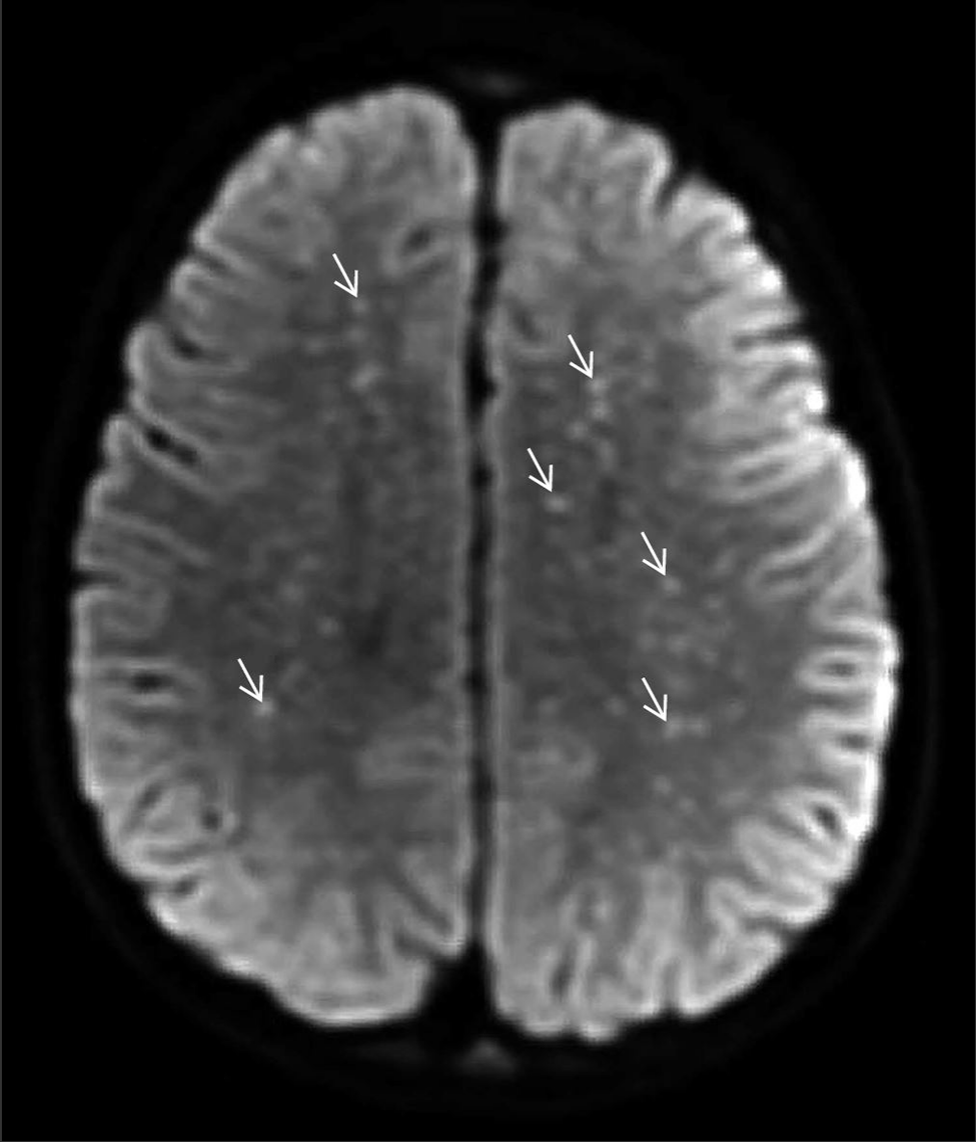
Diffusion-weight magnetic resonance imaging of the brain of a previously healthy 5-year-old boy from Tecate, Mexico, who had Rocky Mountain spotted fever and was treated at Rady Children’s Hospital, San Diego, California, USA. The “starry sky” appearance shows numerous punctate foci of cytotoxic edema involving the supratentorial white matter (arrows), in keeping with acute to subacute injury from small vessel vasculitis.
